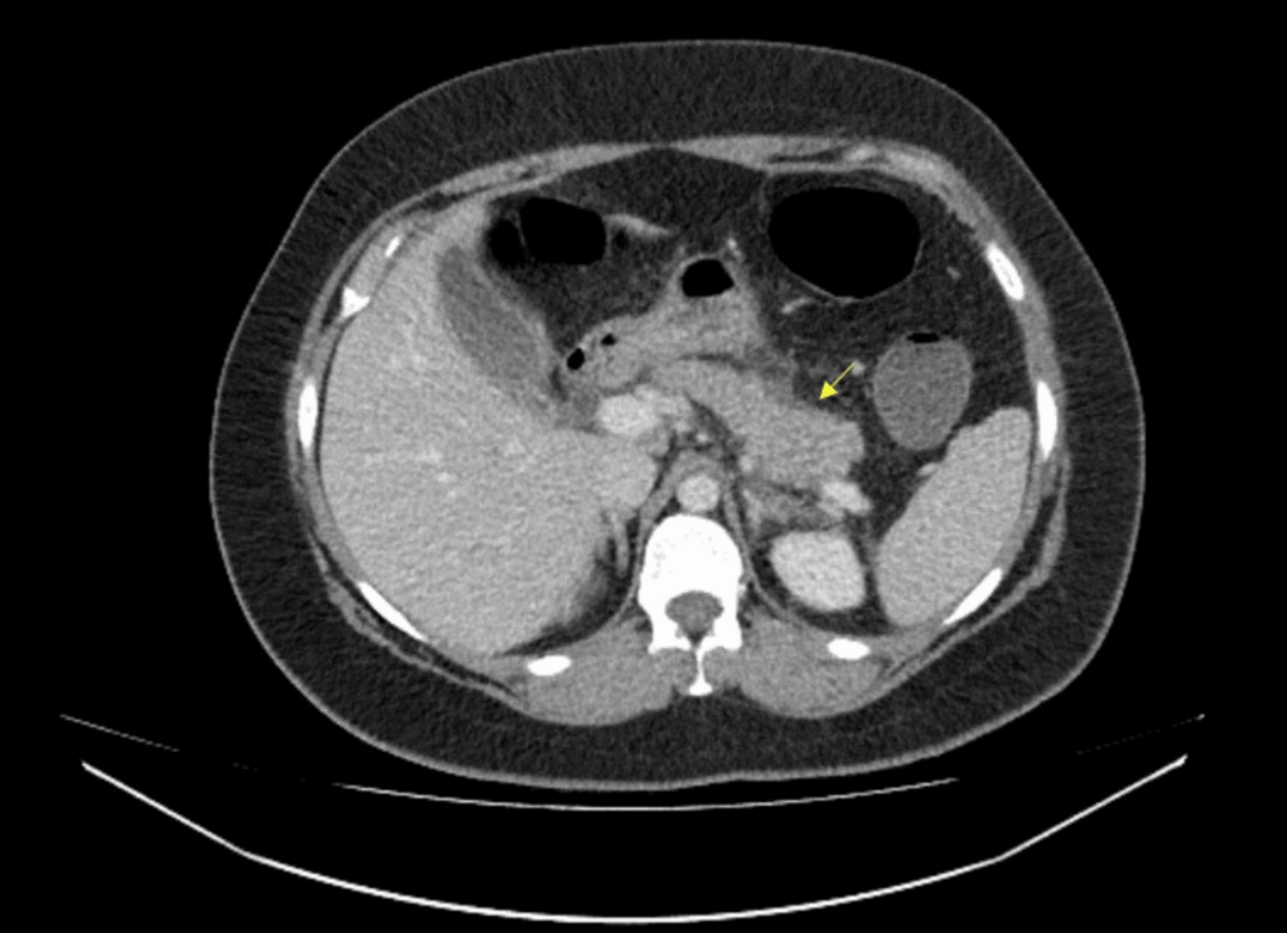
CT Abdomen and Pelvis with contrast The distal head and tail are bulky with peripancreatic inflammatory changes and peripancreatic fluid. Imaging features are in keeping with mild acute interstitial oedematous pancreatitis and mild ascites.
